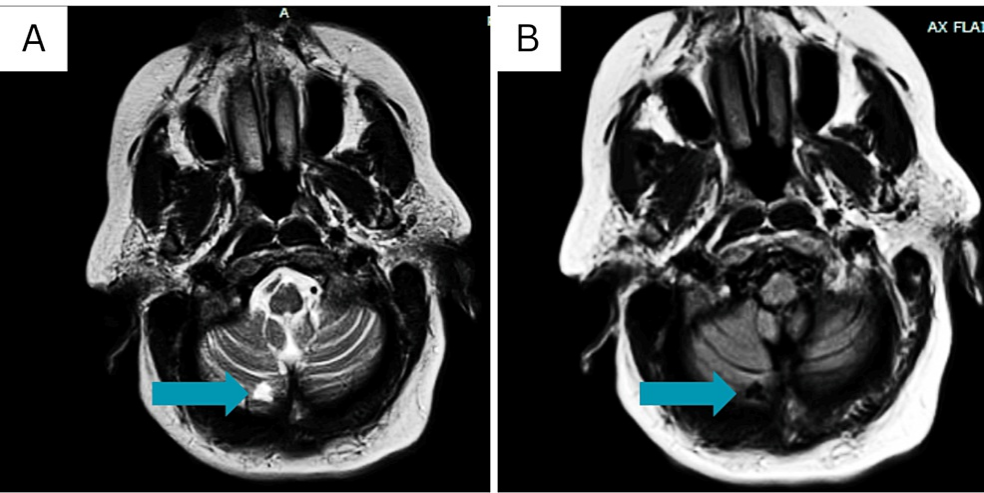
The MRI T2 (A) and FLAIR (B) show an acute right tiny cerebellar infarct (blue arrow). MRI: magnetic resonance imaging; T2: transverse relaxation time; FLAIR: fluid-attenuated inversion recovery
